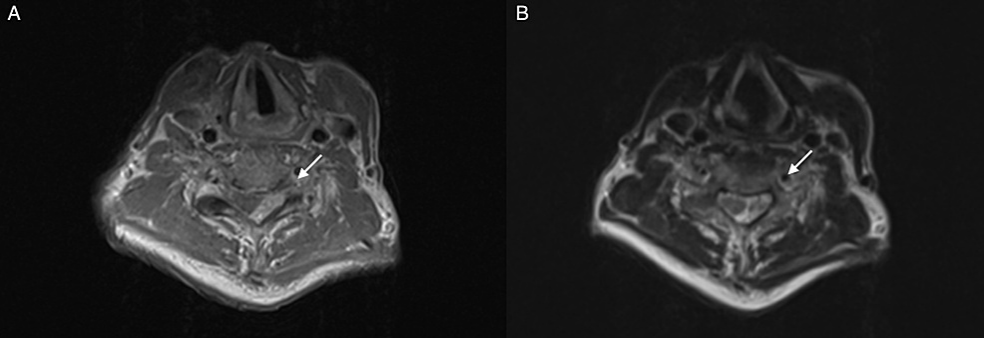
Axial MRI of the spine. (A) T1-weighted axial MRI shows an extramedullary tumor on the left side of the spinal cord causing compression and displacement to the right. (B) T2-weighted axial MRI shows an extramedullary tumor with enhancement of paravertebral soft tissues. MRI, magnetic resonance imaging
